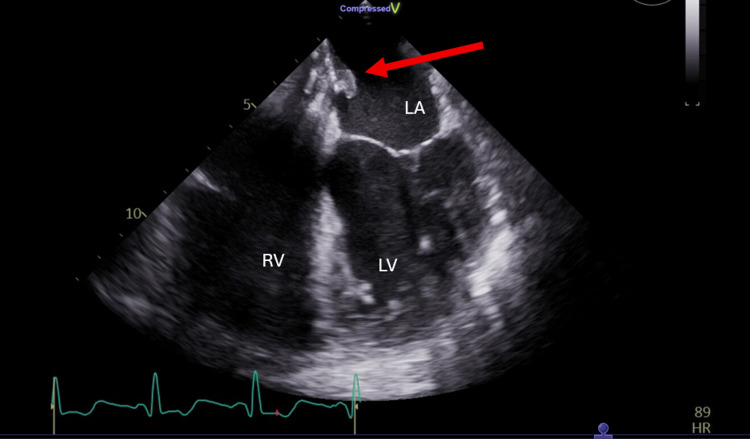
Transesophageal echocardiogram demonstrating a large vegetation adherent to the left atrial side of the atrial septal defect (ASD) occlusion device (red arrow). The device is appropriately positioned within the atrial septum without evidence of device embolization. No valvular pathology, including stenosis, regurgitation, or valvular vegetation, is identified. LA: left atrium; LV: left ventricle; RV: right ventricle
